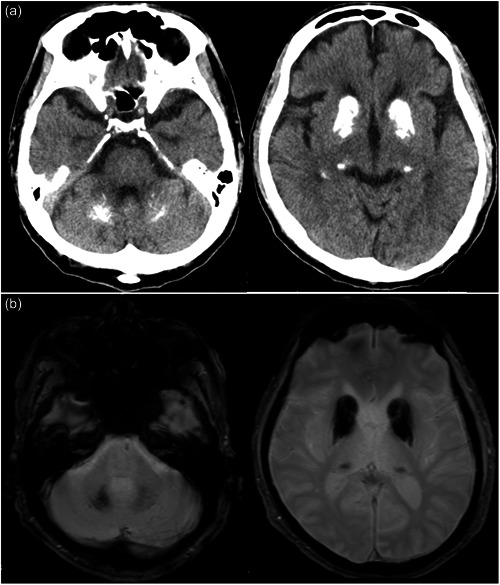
(a) The brain computed tomography (CT) scan revealed symmetrical calcification in the basal ganglia, thalamus, and dentate nucleus, with the extent of calcification slightly larger than before. (b) T2‐star‐weighted magnetic resonance imaging showed calcification in the same areas as did the CT scan.
